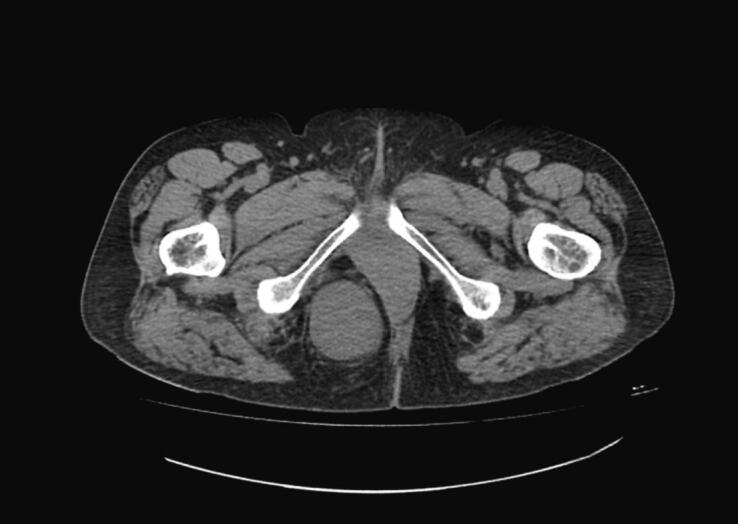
CT shows an 8,5 × 5,5 × 10 cm bilobed mass in the right ischiorectal fossa, extending into the right gluteal region.
